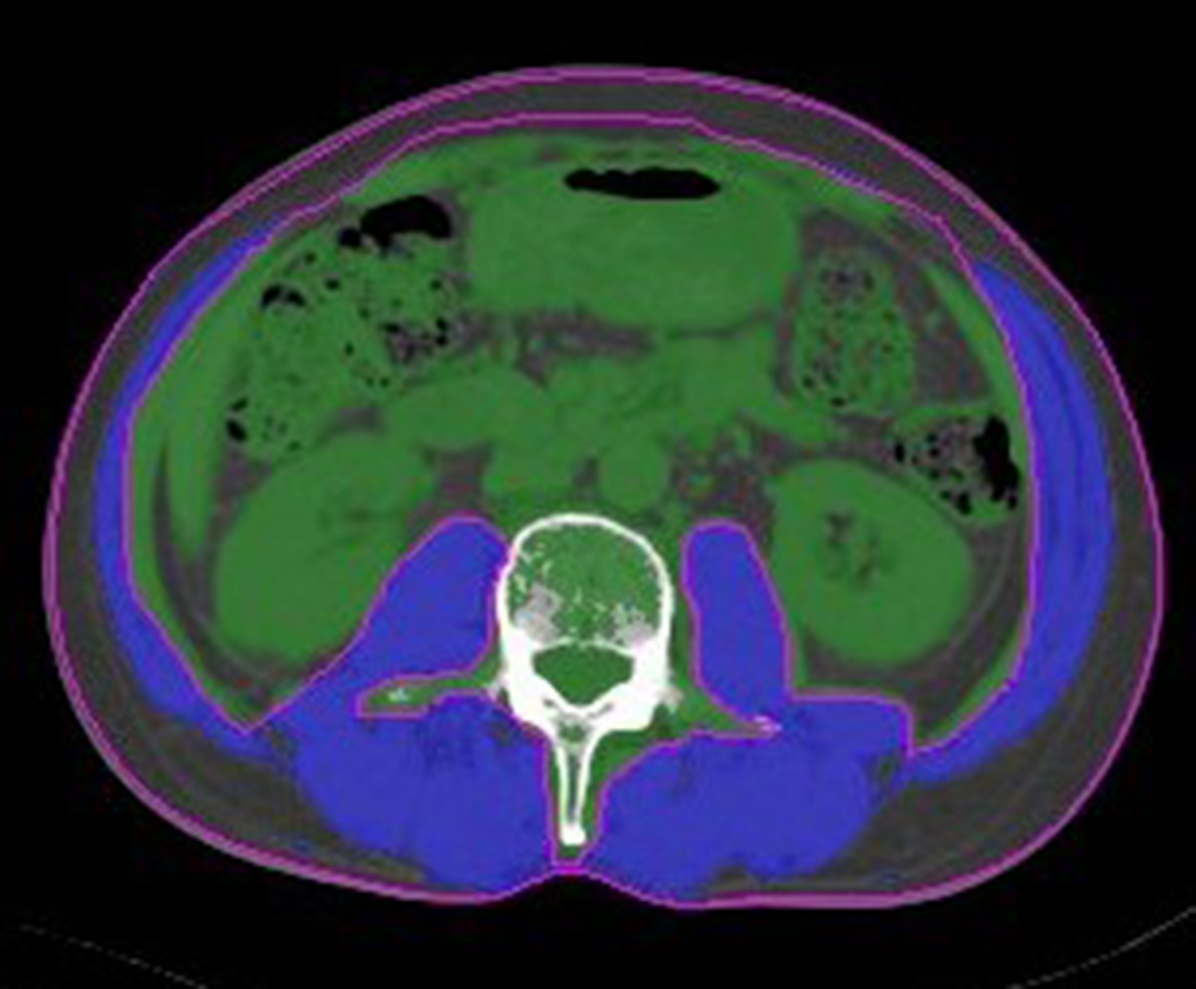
Two adjacent axial CT images of 5-mm slice thickness at the third lumbar vertebrae were used to analyze total muscle cross-sectional area (cm^2^) for each patient. The total muscle cross-sectional area (blue area in the figure) included the abdominal oblique, rectus abdominus, quadratus lumborum, psoas, and erector spine.
